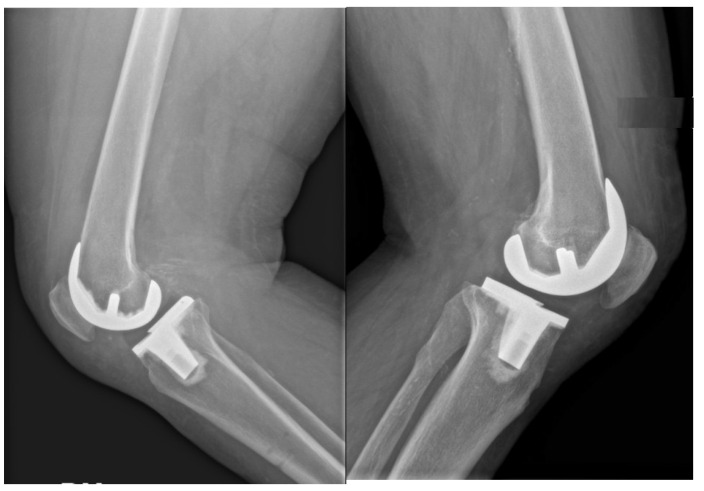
Example of a lateral 90° flexion knee radiograph used for measurements.
